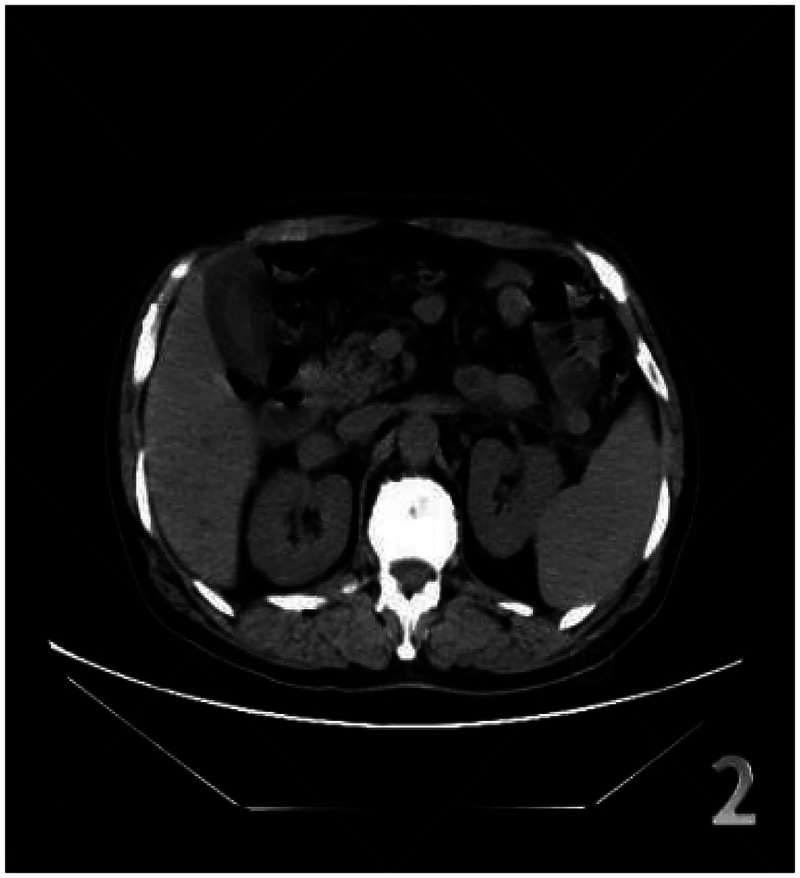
CT revealed increased gallbladder density.
